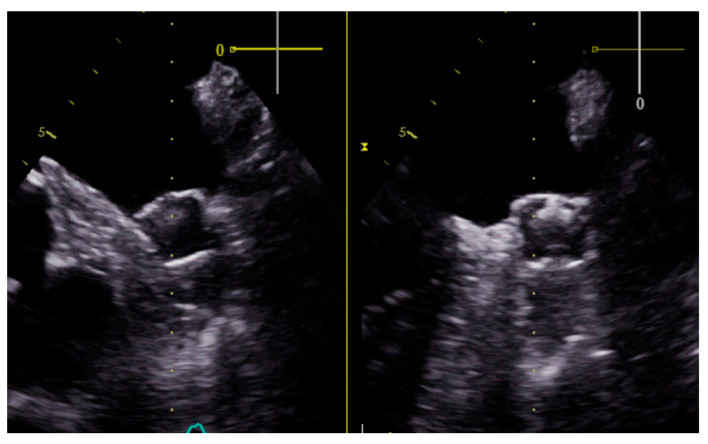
Transesophageal view of a Watchman FLX device in one of our patients three months after the left atrial appendage closure. The procedure was successfully completed, with complete exclusion of the appendage without complications or leaks exceeding 5 mm.
